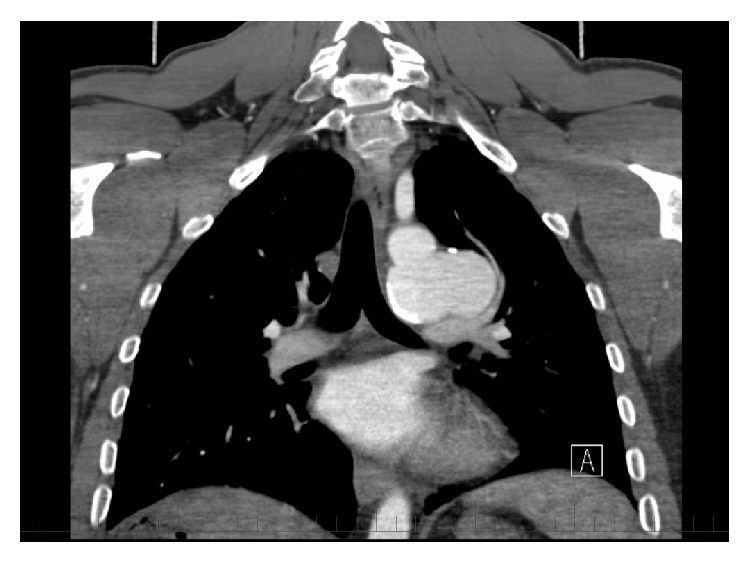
Coronal computed tomography imaging. A 39 mm × 54 mm × 32 mm saccular aneurysm can be seen arising from the aortic isthmus.
